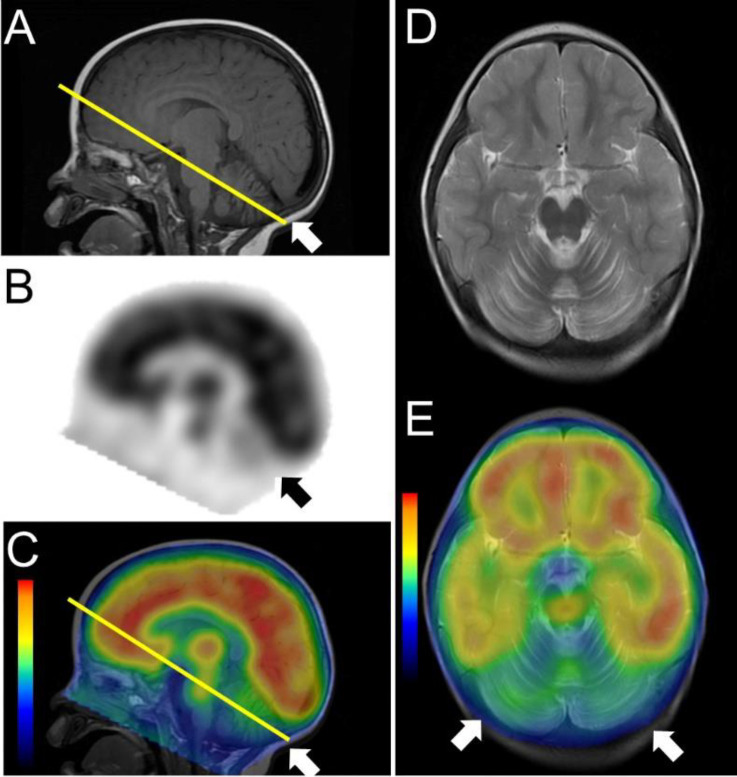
The entire cerebellum showed severe atrophy on Brain MRI (A), and much lower uptake than that in the cerebral cortex on perfusion SPECT and corresponding fused SPECT/MRI (B-E)
